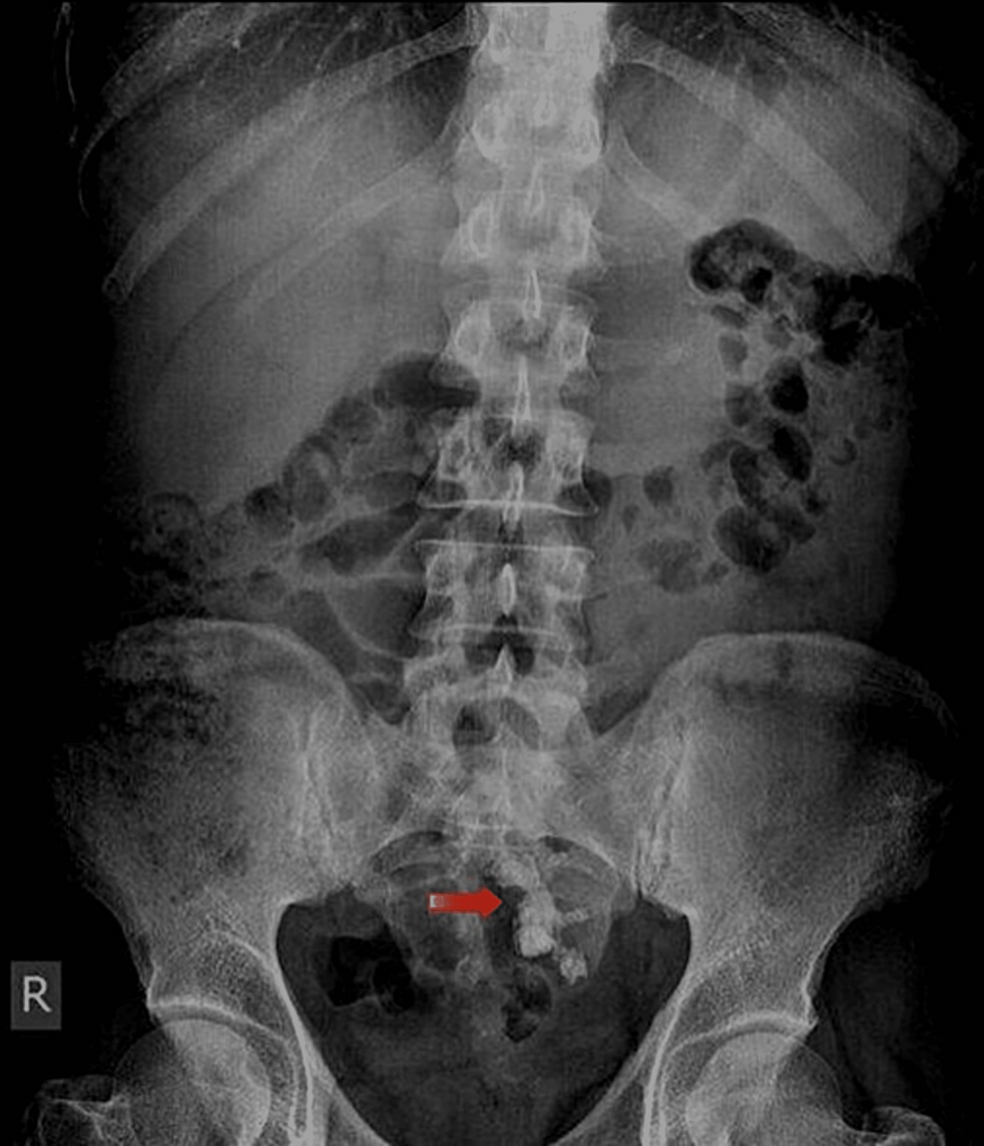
KUB x-ray demonstrating a shadow of a staghorn stone in the pelvic region KUB - Kidney Ureter Bladder
